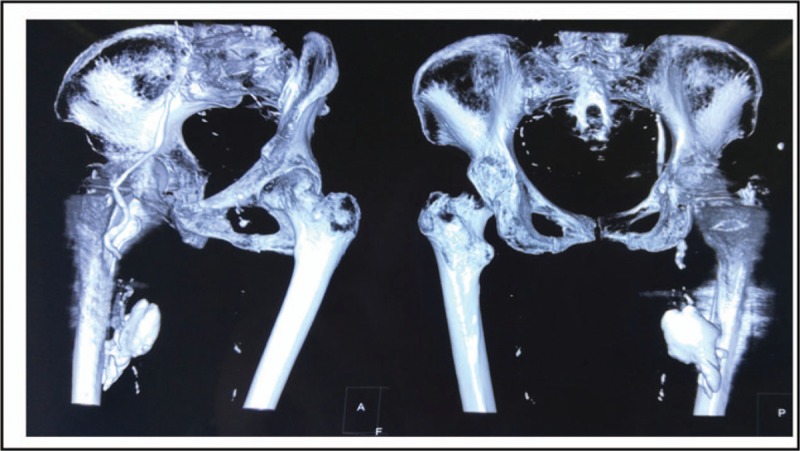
Postoperative 3-dimensional computed tomography reconstruction images. Femoral cortex is consecutive. The leaked bone cement extends to the pelvic cavity.
